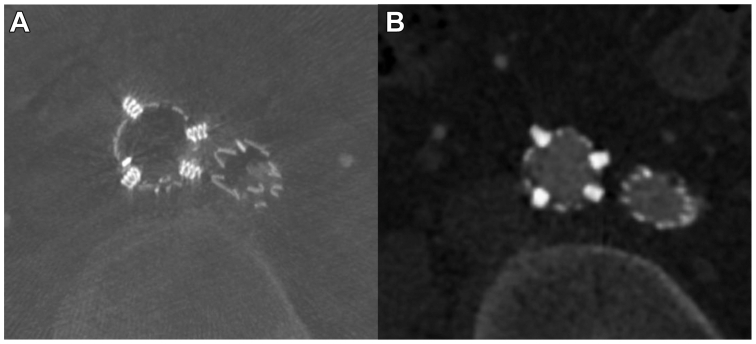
A, Cone beam computed tomography (CT) with CO_2_ performed at the end of the procedure shows the correct placement of the endoanchors. (B) Six months follow-up angio-CT scan confirms the correct placement with no evidence of migration and no signs of endoleak.
